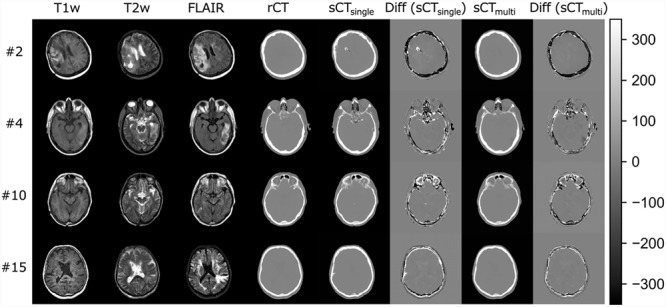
Quantitative comparisons of axial slices between the real and synthetic CT images (rCT and sCT, respectively). Each row shows images of a different representative patient. The sCT images generated from only the T1w sequence (sCT_single_) and from the T1w, T2w and FLAIR MR sequences (sCT_multi_) are shown. The difference maps show the differences in CT number between the rCT and sCT images (sCT—rCT) according to the color bar on the right.
